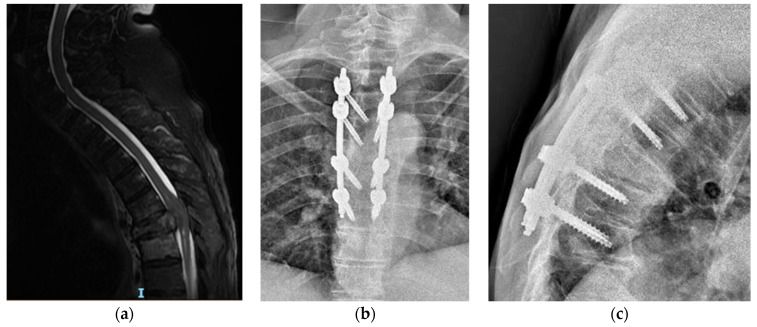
Case of open screw fixation. A 53-year-old female was accepted by our ER because of dorsal pain without any trauma. In her medical history, she recorded breast carcinoma, having been diagnosed 2 years ago and treated with surgery and chemotherapy. She missed regular follow-up appointments. In MRI scan images, we see (a) a lesion involving posterior elements (SINS 13), but without any cord compression. The patient underwent posterior open arthrodesis in order to stabilize the lesion (b,c).
